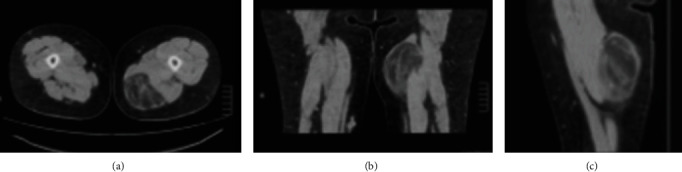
Computed tomography (CT) scan demonstrated 11 x 8 x 7 cm, in vertical x transverse x AP diameter of well-defined, fatty lesion with thick streak fibrous tissue at the right posteromedial of thigh: (a) axial view, (b) coronal view, and (c) sagittal view.
